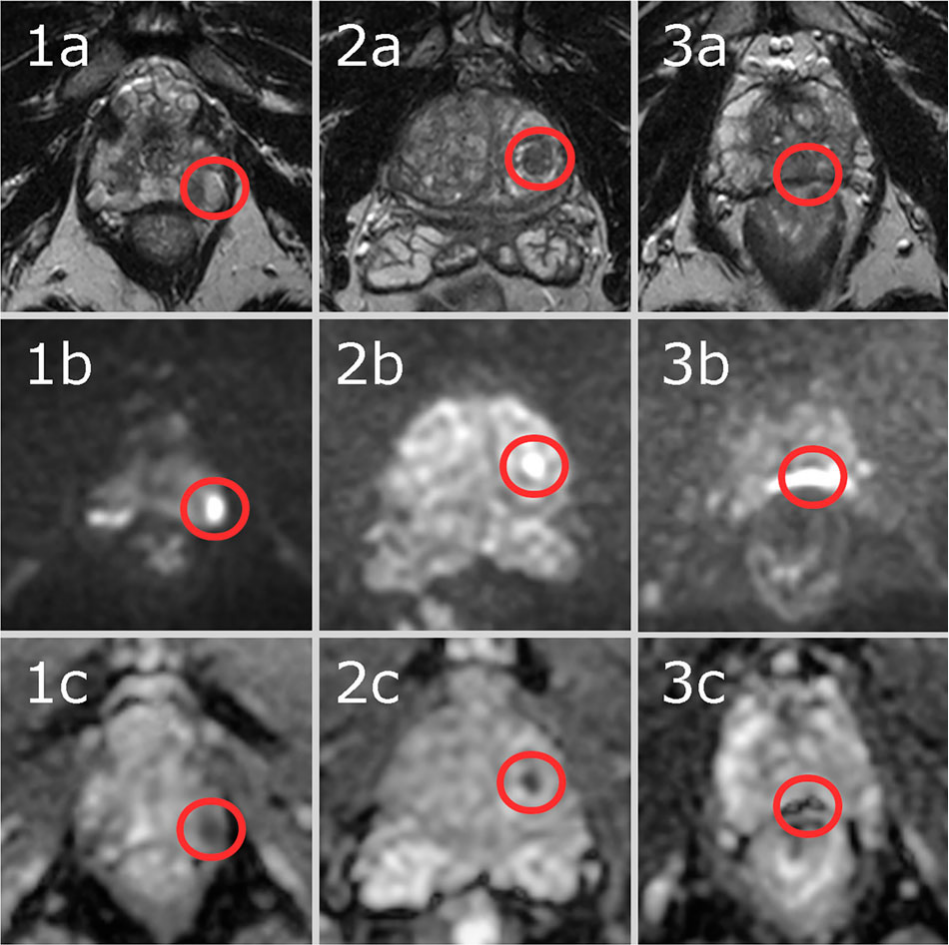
Three example cases (case 1, 2 and 3) from the test set with a negative reference (clinically significant prostate cancer not present), false-positive outputs by the AI system and true negative primary MRI assessment by radiologists. These three cases were selected to represent typical cases of false-positive AI outputs. For each case, a T2-weighted image (a), a high b-value image (b) and an apparent diffusion coefficient map (c) cropped around the prostate are shown from the same slice centered over the AI system’s output. The AI system’s findings are marked with a red circle surrounding the area instead of the segmentation, to improve visibility of the findings. Case 1. Man with PSA 3.0 ng/mL and a prostate volume of 33 mL resulting in a PSA-density of 0.09 ng/mL^2^. Systematic biopsies were performed and showed benign results. The AI system marked a lesion in the left apical part of the prostate. In the area, a periprostatic vein with markedly restricted diffusion can be seen. Case 2. Man with PSA 13.6 ng/mL and a prostate volume of 91 mL resulting in a PSA-density of 0.15 ng/mL^2^. No biopsies were performed. The AI system marked a lesion in the left transitional zone. In the area, a well-circumscribed lesion in the transitional zone can be seen with markedly restricted diffusion. Case 3. Man with PSA 2.3 ng/mL and a prostate volume of 30 mL resulting in a PSA-density of 0.08 ng/mL^2^. No biopsies were performed. The AI system marked a lesion in the middle dorsal part of the prostate. In the area, severe pile-up artifacts on the diffusion-weighted sequence caused by gas in the rectum can be seen
